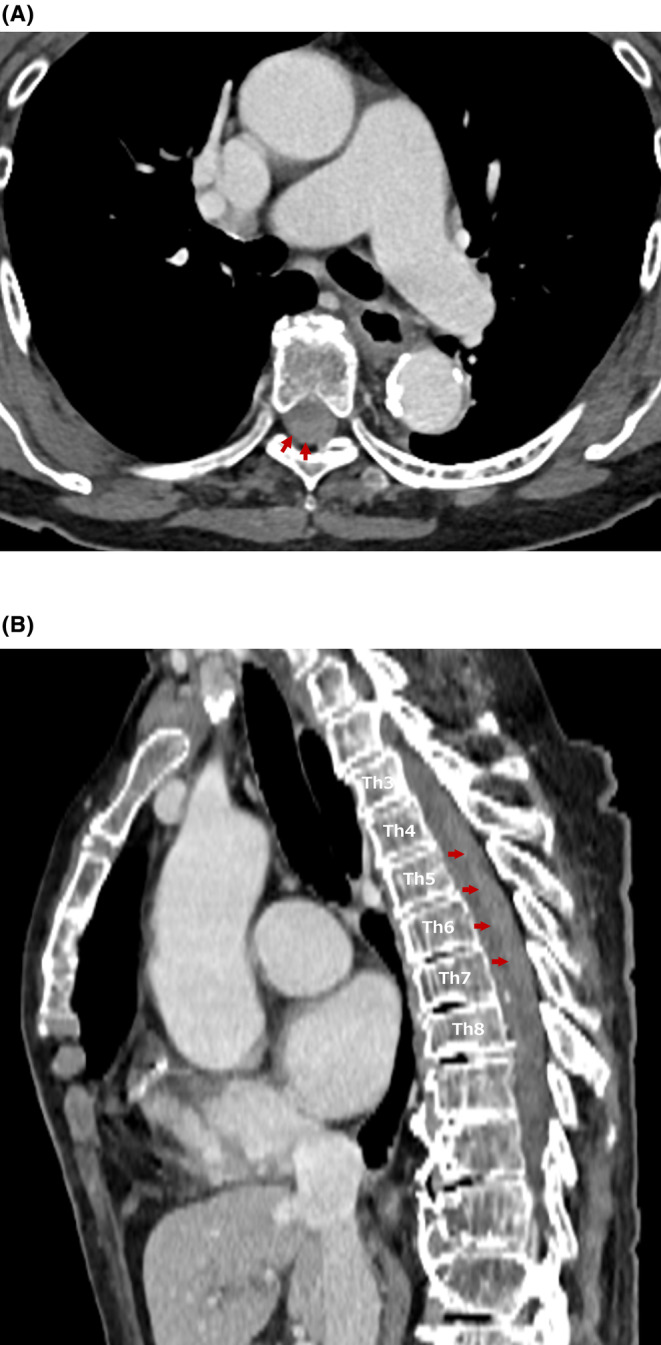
Axial and sagittal computed tomography. (A) Axial contrast‐enhanced computed tomography of the thoracic spine reveals a spinal epidural hematoma at six vertebral levels (red arrows). (B) Sagittal computed tomography reveals a spinal epidural hematoma from thoracic vertebra 2–7 (red arrows).
